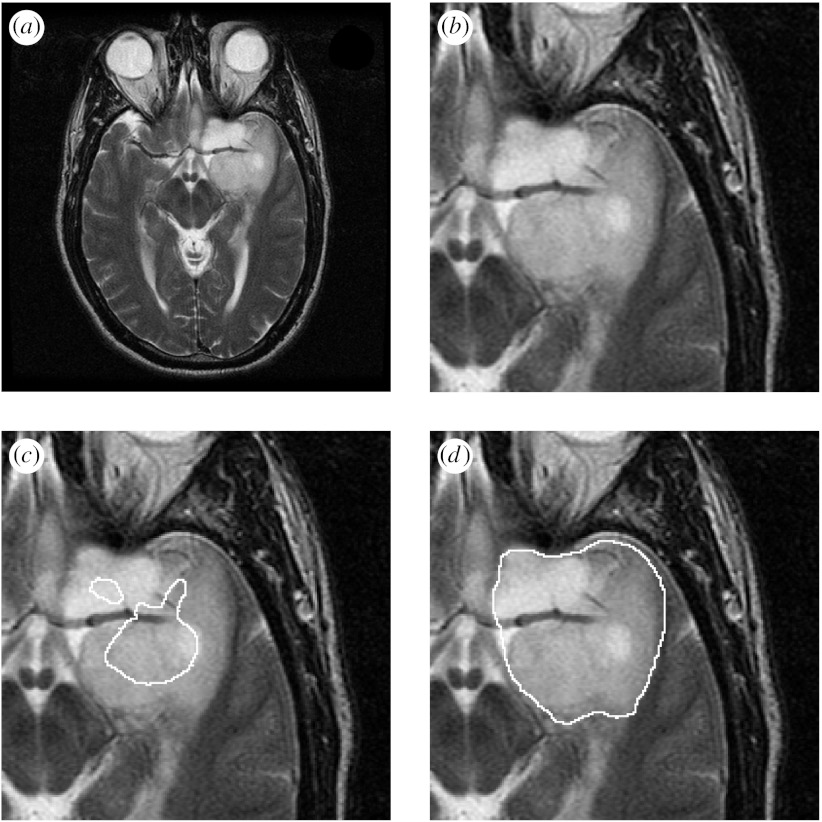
Average and estimated true tumour segmentations from human rater segmentations, binarized by thresholding the estimated true score at 0.0. (*a*) MRI of a brain tumour. (*b*) Region of interest. (*c*) Binarized average of rater segmentations. (*d*) Binarized estimated tumour segmentation.
